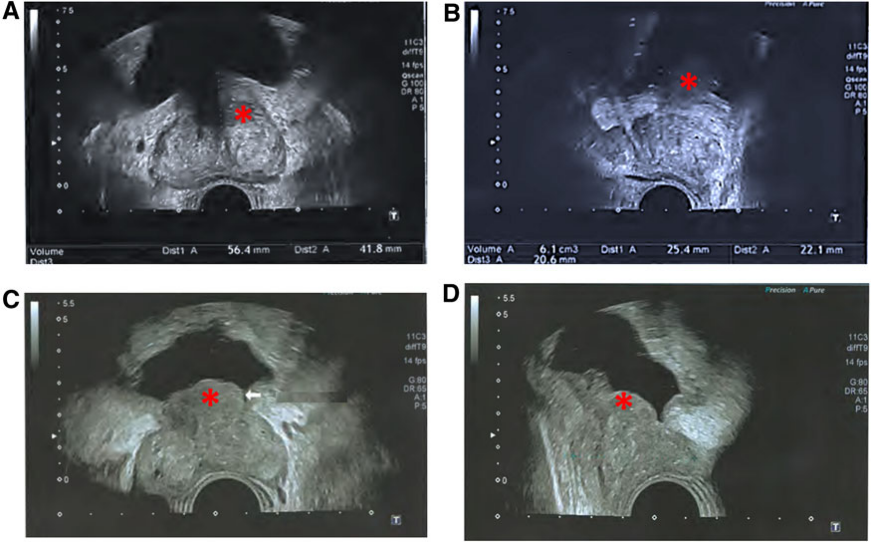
TRUS examination before (A, B) and after surgery (C, D). This picture represents the face (A, C) and side (B, D) of prostate, on TRUS examination before GreenLight photovaporization (A, B) and after (C, D). We find a 62 g prostate with median lobe protruding into the bladder (*) before surgery that stayed after with a major decrease of prostate volume (30 g). TRUS, transrectal ultrasound.
